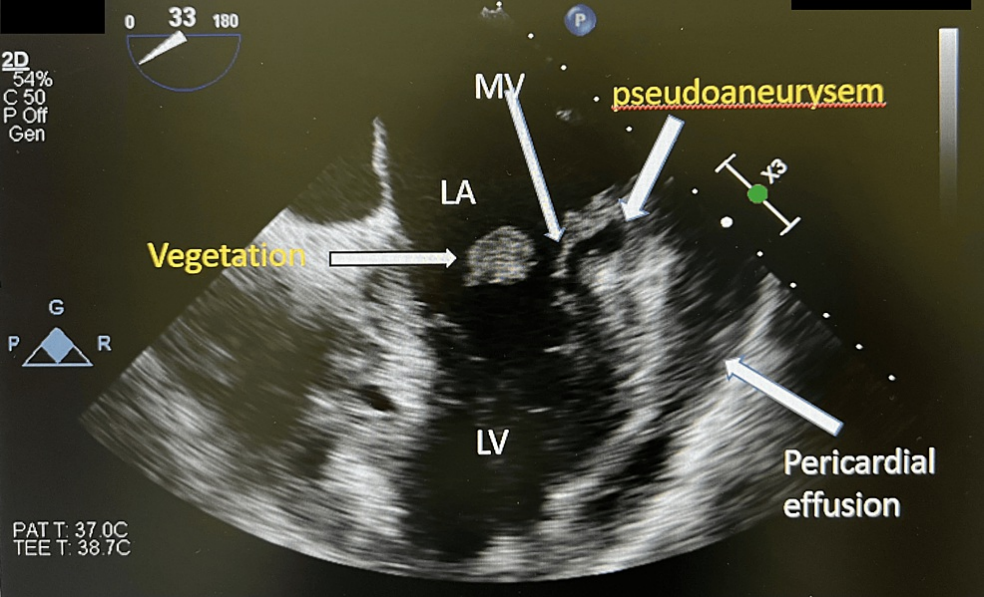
Echocardiography in Case 3 revealed vegetation with evidance of pseudoaneurysem.
